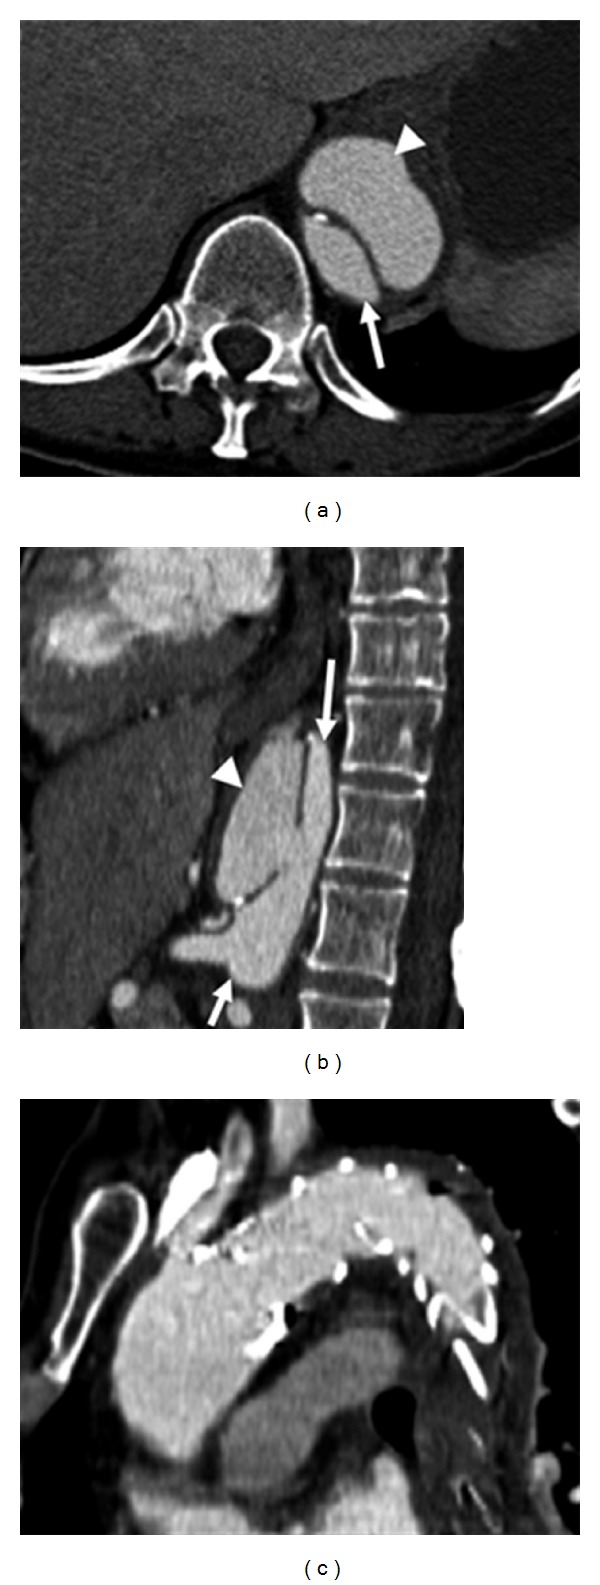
(a) CTA after three weeks after first intervention shows a newly developed dissection extending to the celiac trunk (arrow: true lumen and arrowhead: false lumen). (b) Sagittal reconstruction of the same CTA shows wide communication between the true and false lumina (arrows: true lumen and arrowhead: false lumen). (c) Coronal CTA reconstruction showing the satisfying position of the primary implanted stent graft.
